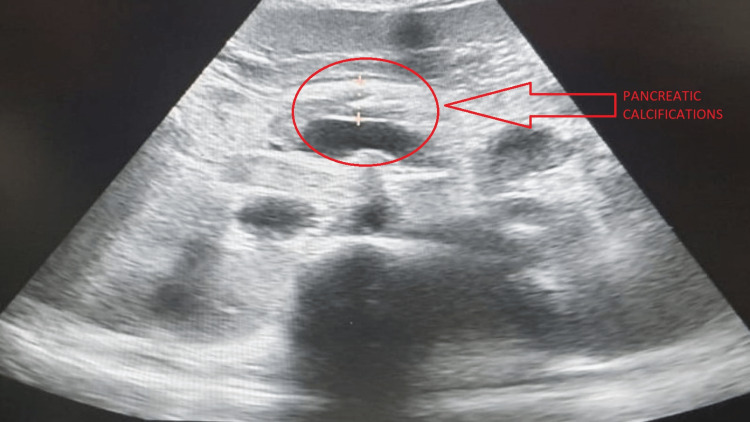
Ultrasonography image showing pancreatic calcifications
